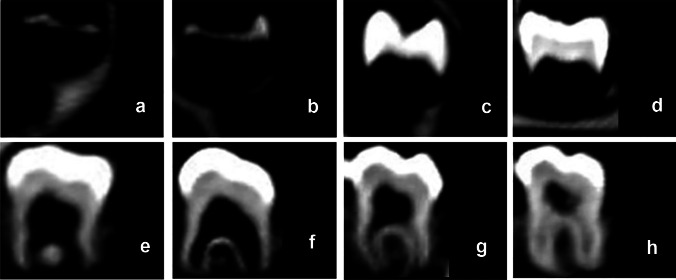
Computed tomography images depicting the process of permanent tooth maturation, classified based on Demirjian’s method [2, 12]. a Stage A—beginning of calcification (no fusion of single calcified occlusal points). b Stage B—calcification points fuse, the contour of the occlusal surface is recognizable. c Stage C—enamel formation has been completed, dentin formation has begun. d Stage D—crown formation is complete. e Stage E—root formation, the radicular bifurcation forms in molars. f Stage F—the root length is equal to or greater than the crown height. g Stage G—the roots have almost reached their full length, the apical end is partially open. h Stage H—the root apex is completely closed; image source: in-house archives
